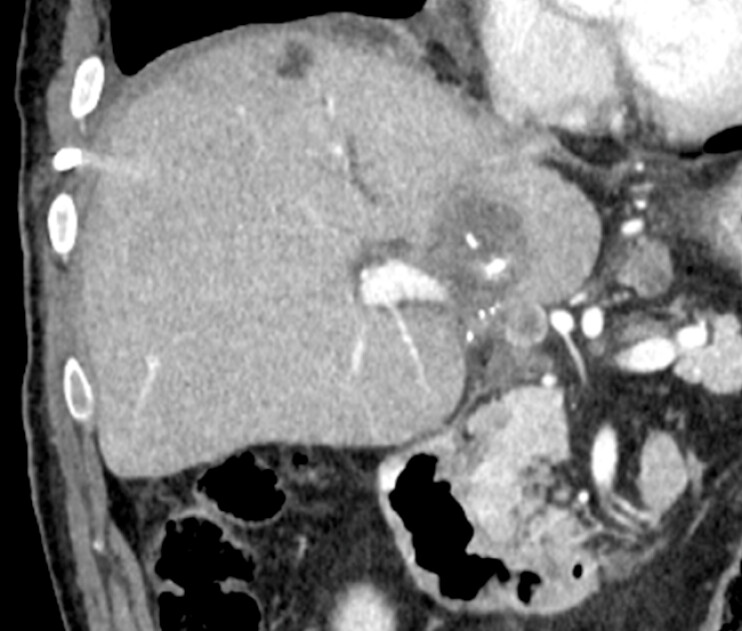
Contrast-enhanced CT revealed recurrent hepatocellular carcinoma in the liver S1. CT, computed tomography.
